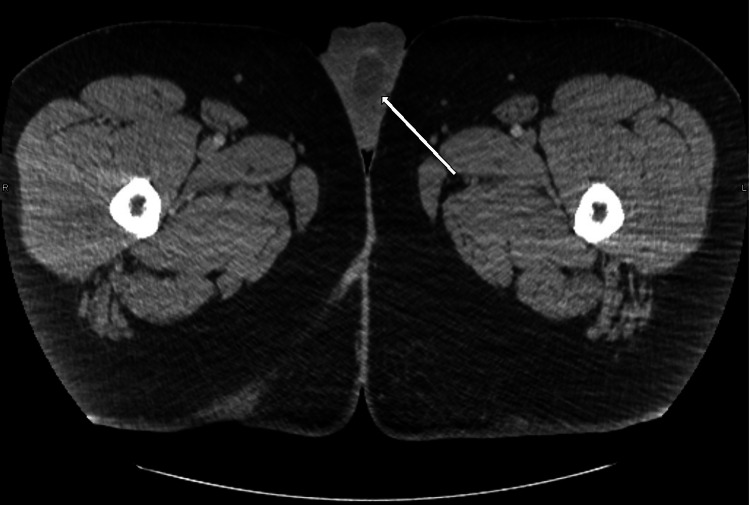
CT of the scrotum showing a left testicular mass (white arrow). CT: computerized tomography
